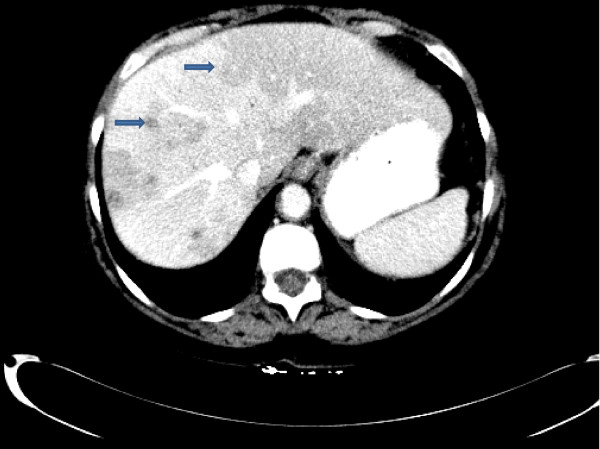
Computed tomography (CT) scan showing multiple low attenuation liver lesions.
